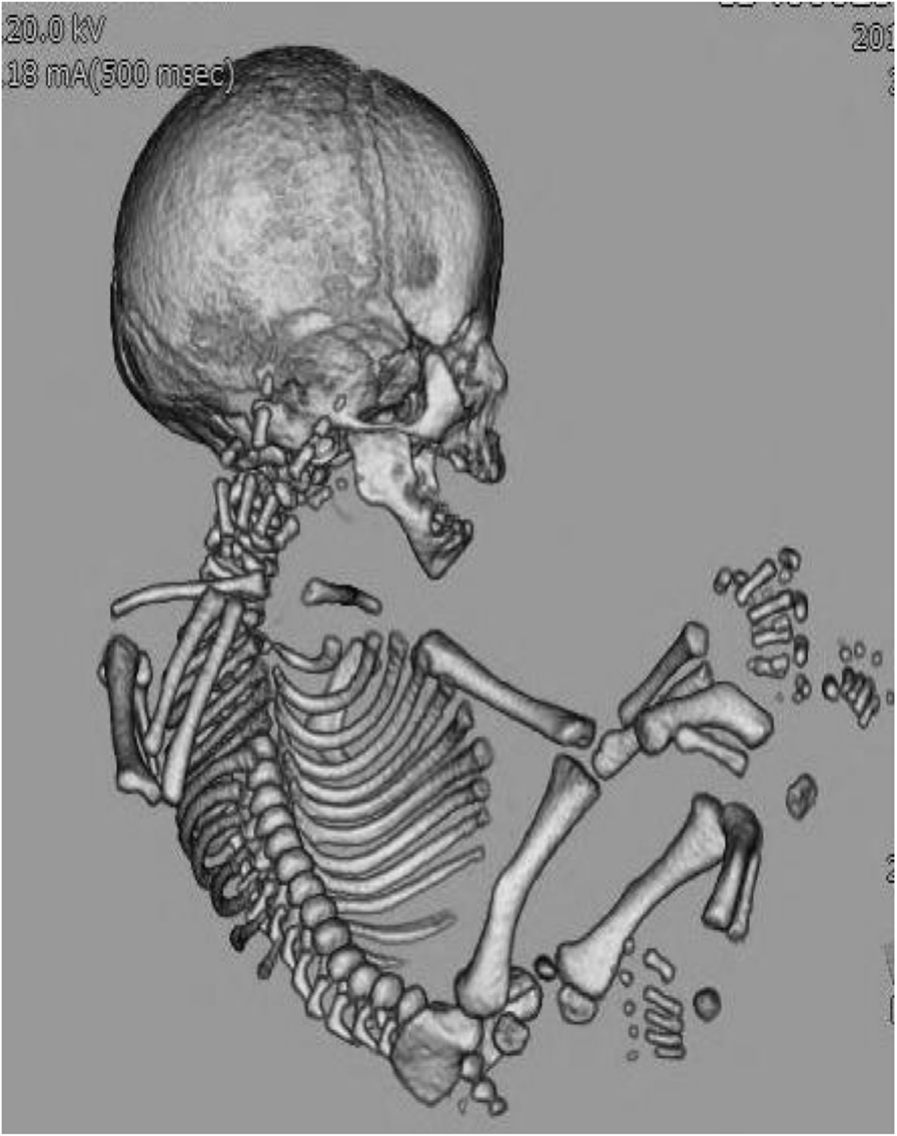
Fetal 3-dimensional computed tomography imaging of Infant 13: A case of campomelic dysplasia with a postnatal diagnosis that was different from the prenatal diagnosis
